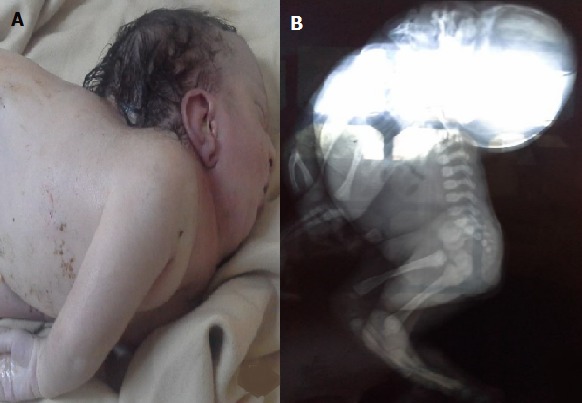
Aspect du nouveau-né avec iniencéphalie; A): tête en hyperextension; B) : radiographie montrant la malformation thoraco-vertébrale, la cyphose rachidienne dorsale et la non visualisation des vertèbres cervicales
